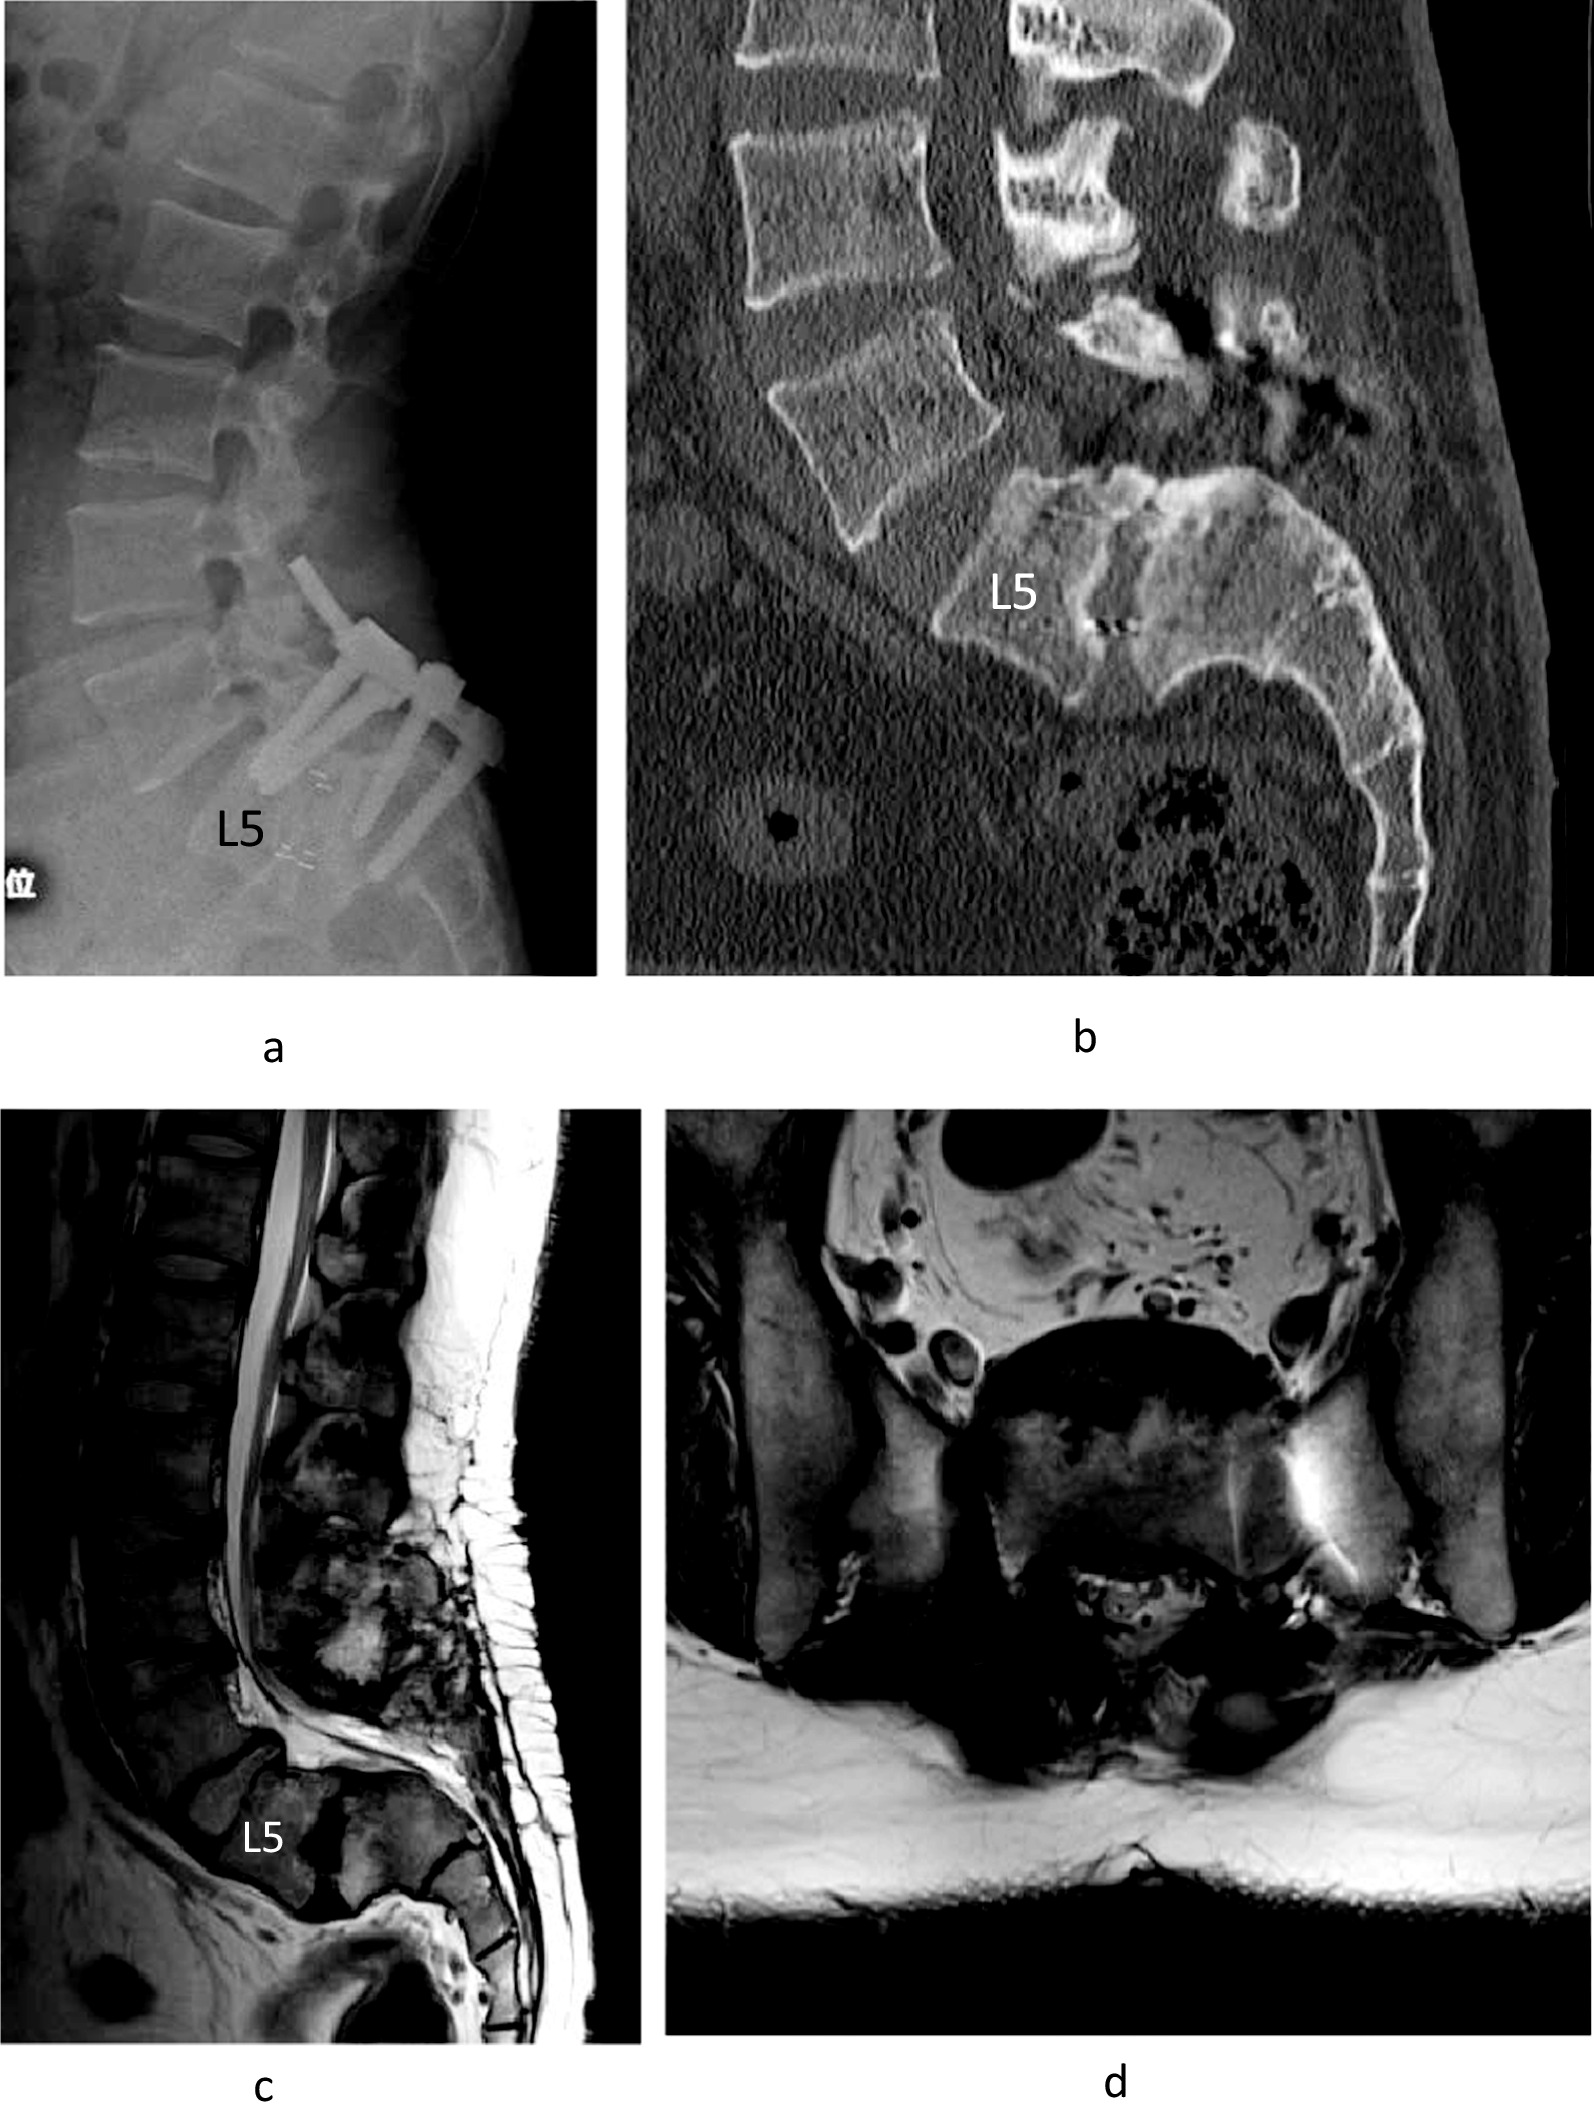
Posterior lumbar interbody fusion with mild reduction of L5/S1 was performed on the older brother with L5 and S1 pedicle screws (a). Computed tomography showed bony fusion between L5/S1 (b). MRI showed that decompression was established (c, d) 5 years after the operation
